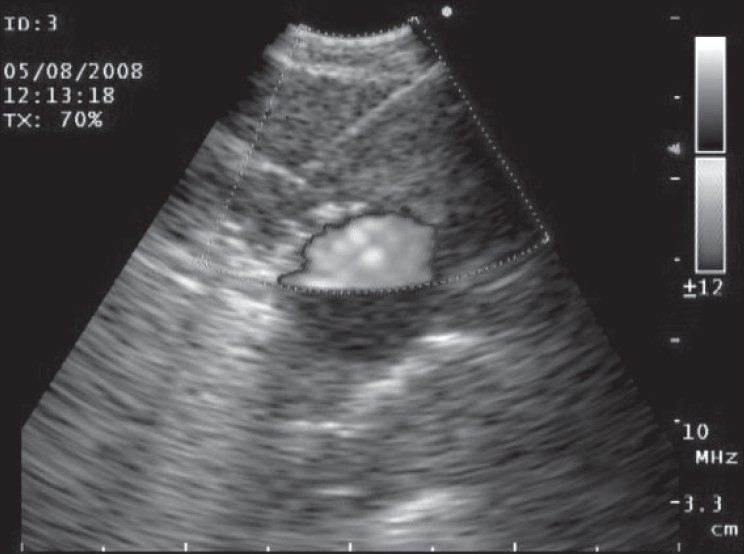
Real-time TBNA. Needle seen inside the lymph node. Doppler showing the blood vessels
